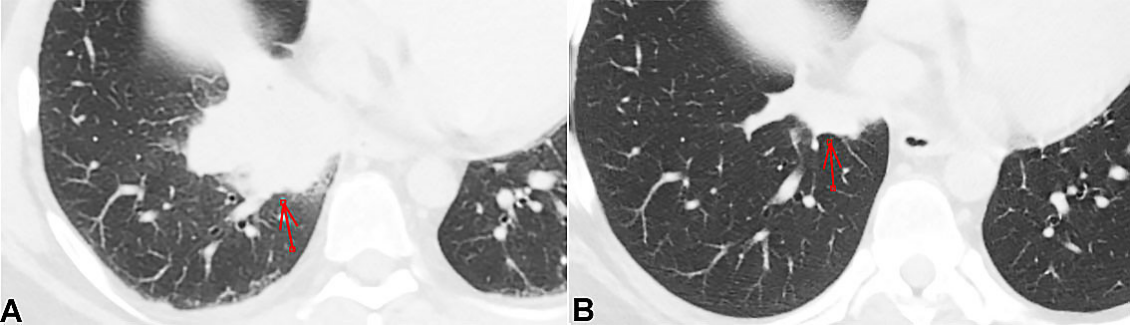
Axial lung window non-enhanced CT scan images of the chest show a significant decrease in the size of the right lower lobar lobulated metastatic mass post stereotactic radiation therapy and a dose of Atezolizumab (B) when compared to the images done prior (A).
